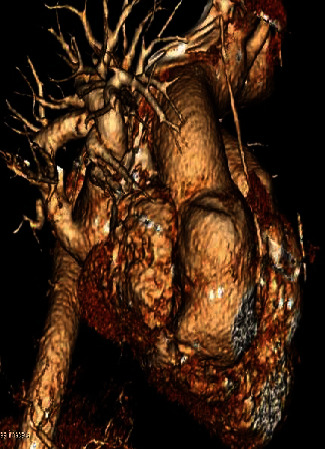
Computed tomography angiography of the sinus of Valsalva aneurysm, sagittal view. The right and noncoronary cusps are fused, separated by a raphe.
